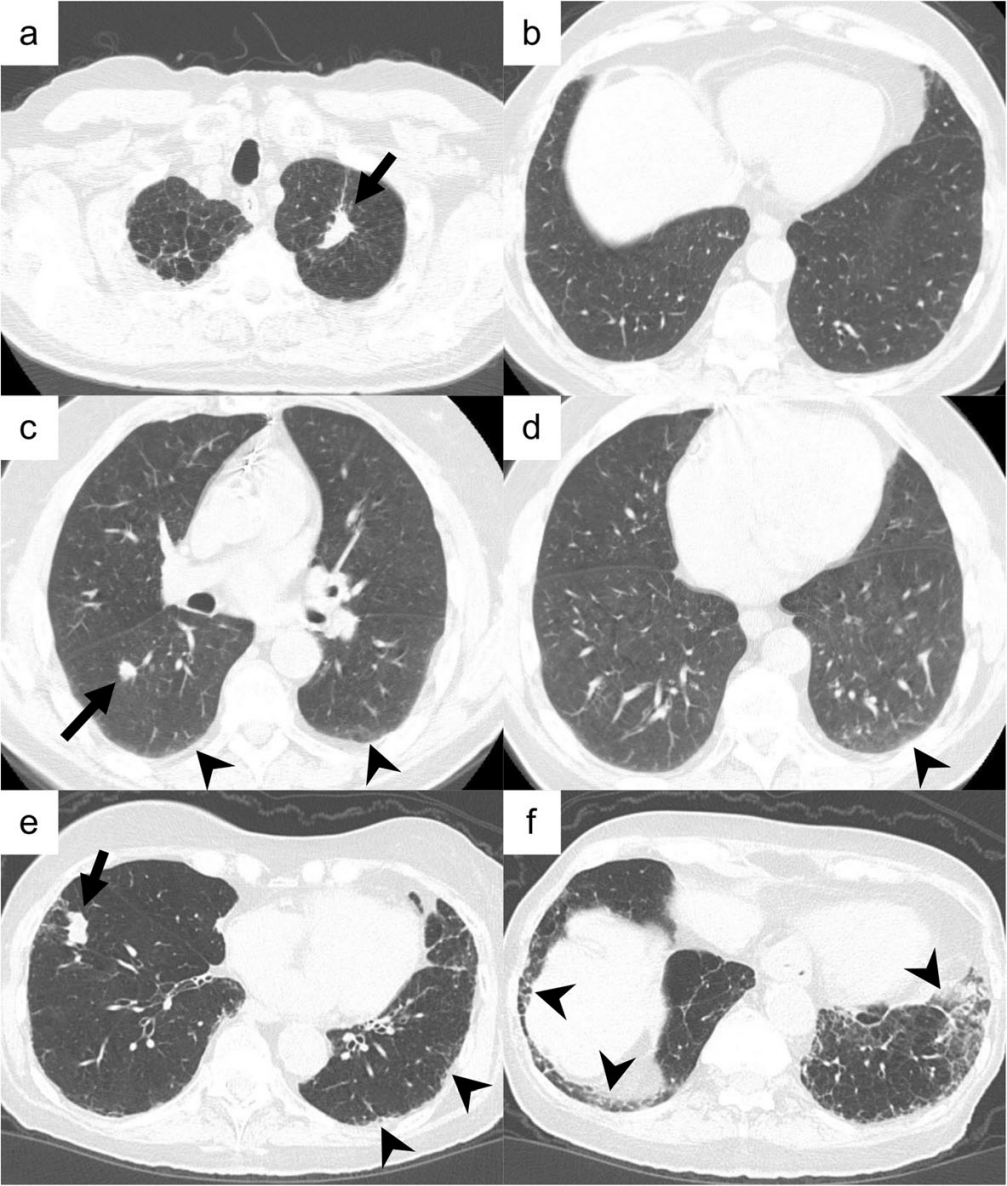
Examples of the ILA scoring. Axial CT images of three patients with lung cancer (arrows). a, b A 74-year-old male affected by squamous cell carcinoma of 2.5 cm in the left upper lobe (arrow). There was no interstitial lung abnormality (ILA; ILA score = 0). c, d A 64-year-old female affected by squamous cell carcinoma of 1.2 cm in the right lower lobe (arrow). The arrowheads demonstrate subpleural ground-glass opacity in less than 5% of the lung. The ILA score was 1 (equivocal for ILA) because it was unclear whether the finding was only dependent opacity or contained ILA. e, f A 84-year-old female affected by squamous cell carcinoma of 2.5 cm in the right lower lobe (arrow). The arrowheads point to subpleural ground-glass opacity and reticulation. These findings were seen in not only the dependent area but also in the ventral side. The ILA score was 2 (considered as ILA definitely)
